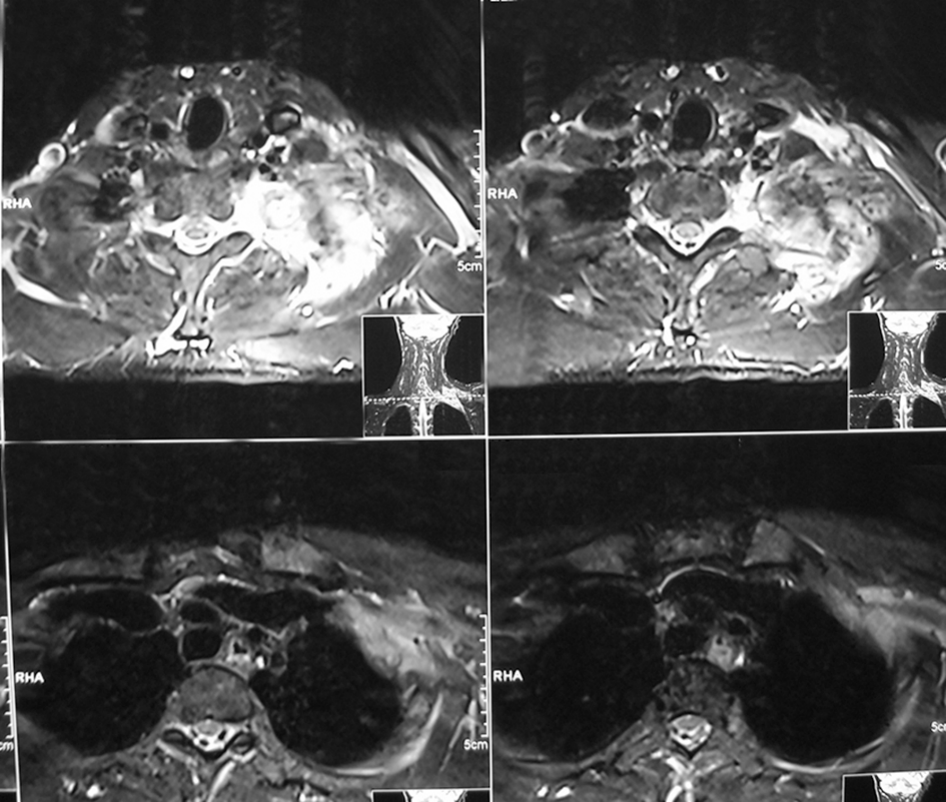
MRI reveals extensive edema around the lesion and high signal intensities on T2WI with punctate low signal areas consistent with mineralization on T1WI.
